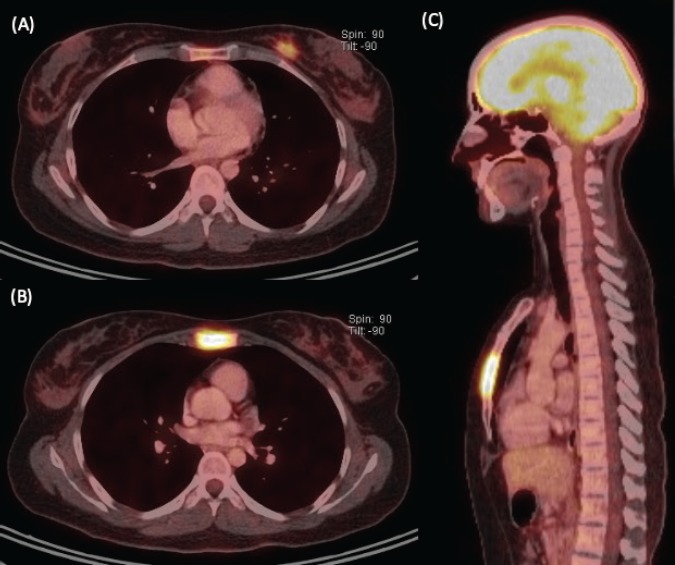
Staging image study. (A) Axial view of PET/CT showing a hypermetabolic left breast nodule of 18 mm at the LIQ, compatible with primary neoplasia. (B) Axial and (C) sagittal views of PET/CT demonstrating extensive substitutive hypermetabolic sternal compromise with cortical osteolysis (maximum SUV 7.7).
